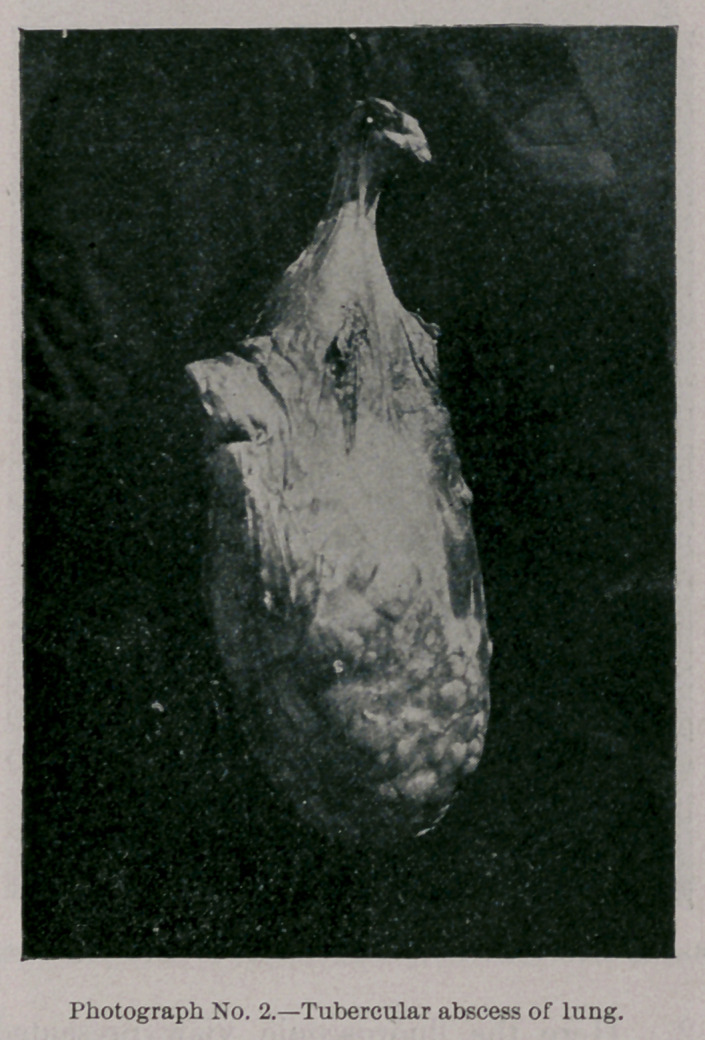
Radiograph No. 2. f4:
